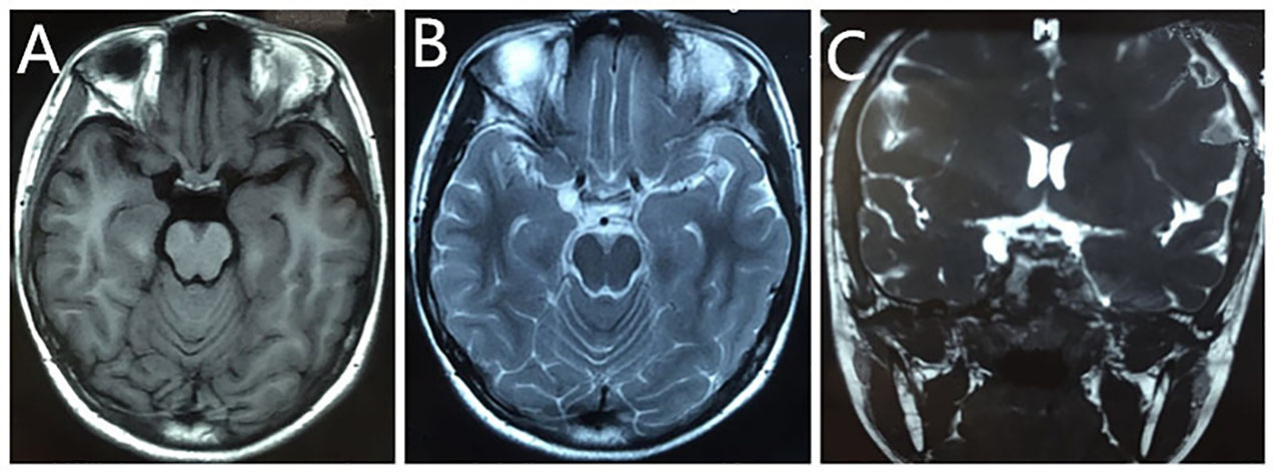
Preoperative MRI scan of the patient. T1-weighted images revealed a well-defined hypointense lesion at the right side of the saddle (A). T2-weighted sequences showed a small cystic and homogeneous hyperintense lesion, measuring approximately 1.0 cm × 1.2 cm (B). Cranial neuroimaging revealed a dumbbell-shaped cystic shadow on the right side of the saddle, suggesting a potential small cyst of the right oculomotor nerve root sheath (C).
